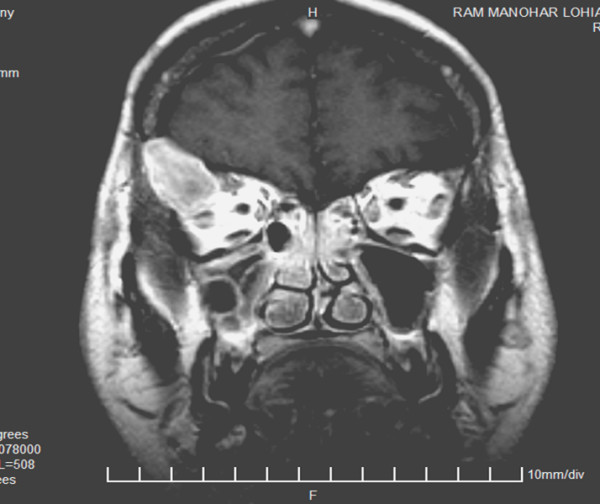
MRI scan showing destruction of right sphenoid, frontal bones and zygomatic arch with associated soft tissue component extending laterally into the infratemporal fossa and medially into the orbit.
